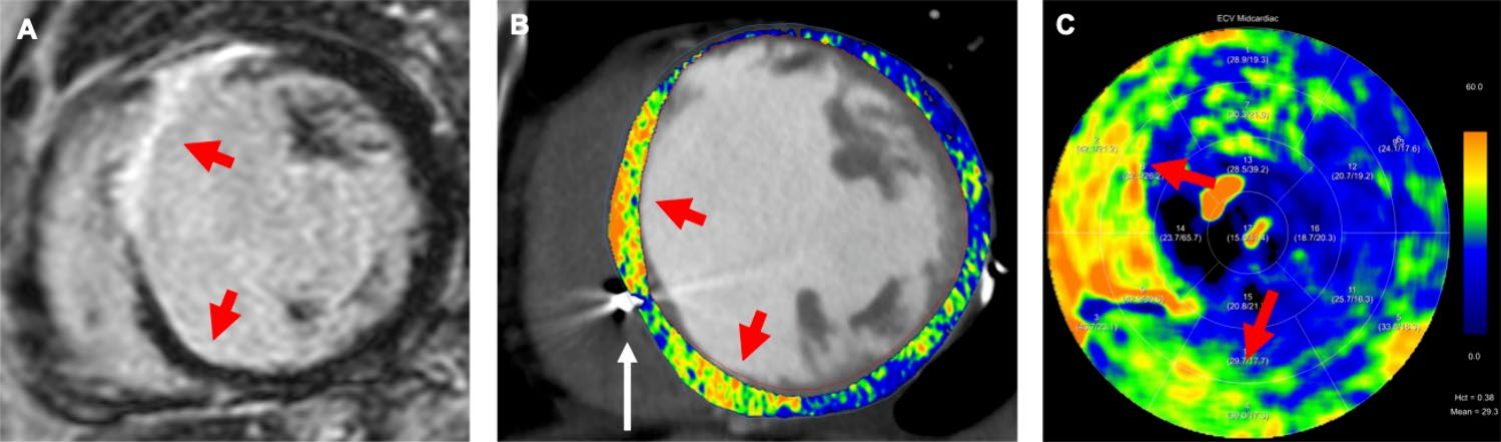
Seventy four-year-old female patient with chronic myocardial infarction after occlusion of the left anterior descending artery. Red arrows indicate antero-septal transmural and infero-septal subendocardial scar in late gadolinium enhancement MRI in the A short-axis view. Extracellular volume overlaid onto coronary CT angiography in the B short-axis and in a C polar map. White arrow shows a pacemaker lead in the right ventricle B inserted after MRI due to arrhythmia
